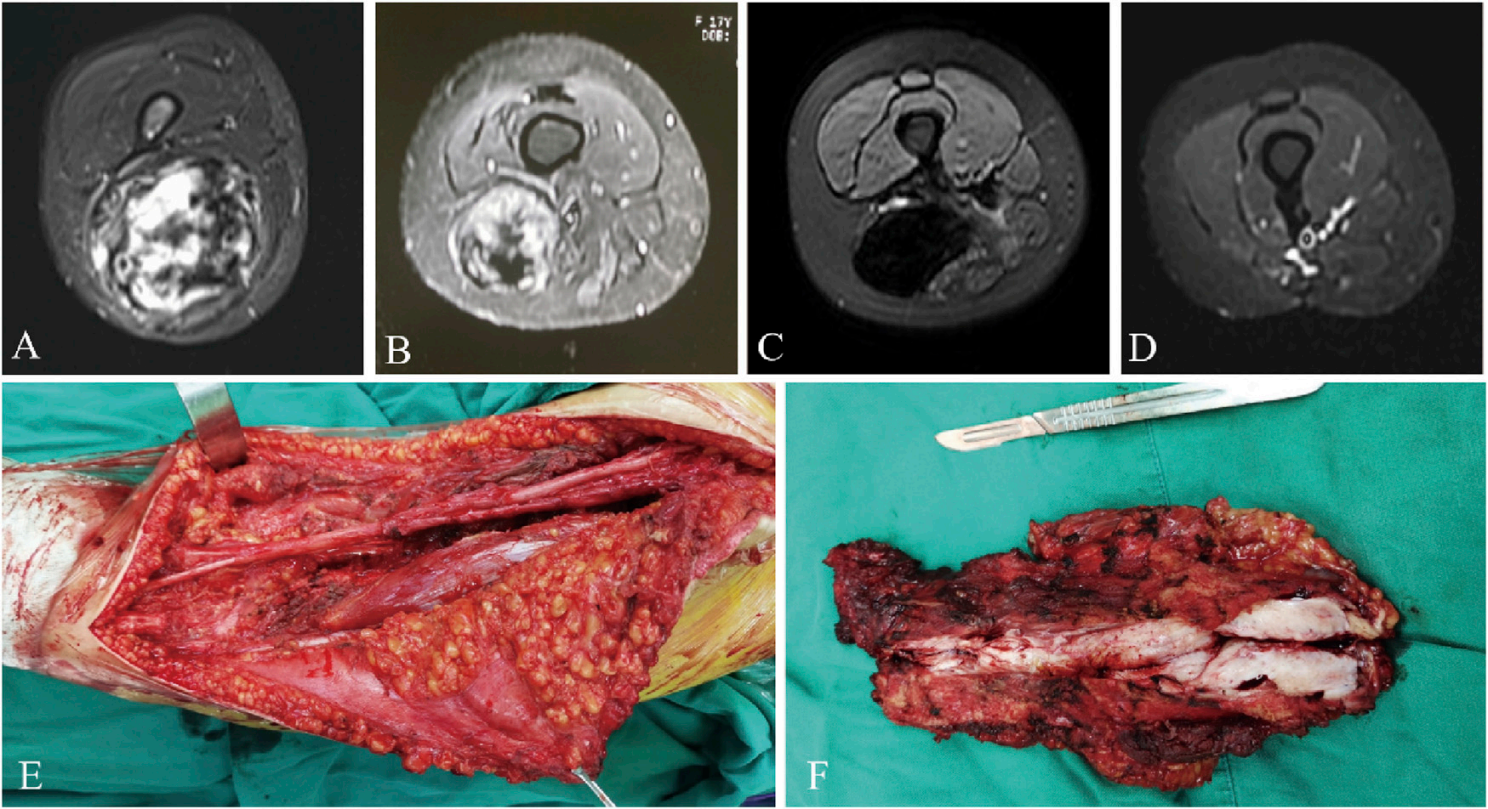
A 20-year-old female presented with postoperative recurrence of DF of right thigh (A), which still recurred 17 months after tumor resection in our hospital (B). MRI was re-examined after using anlotinib (C), and the tumor was resected again with preserved vascular and nerve during the operation (E, F), and MRI was re-performed 14 months after surgery (D). MRI, magnetic resonance imaging.
